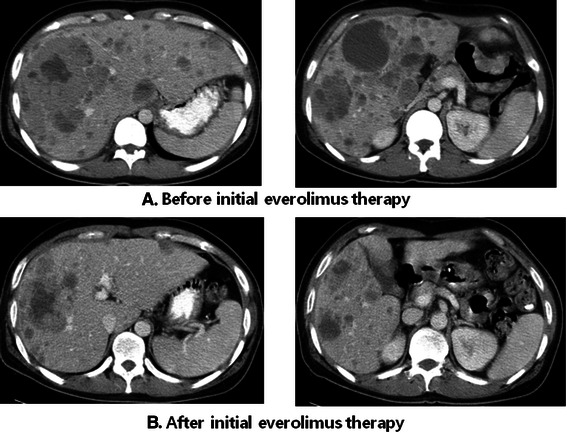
The patient received initial treatment of everolimus 10 mg/d as the third-line chemotherapy. CT scans were taken every 2 months during the initial treatment period. CT scans reveal the diffuse liver metastases before treatment with everolimus (A). After 1 year and 2 months of everolimus 10 mg/d, partial response was achieved (B).
